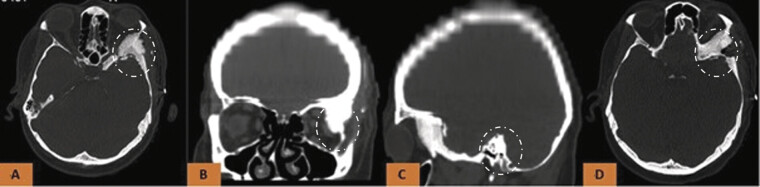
Fibrous dysplasia. An intradiploic, hyperdense, expansile lesion measuring 42 × 32 × 26 mm with a homogeneous ground-glass appearance is noted in the left orbit and zygomatic bone on axial, coronal, and sagittal cranial CT images (
